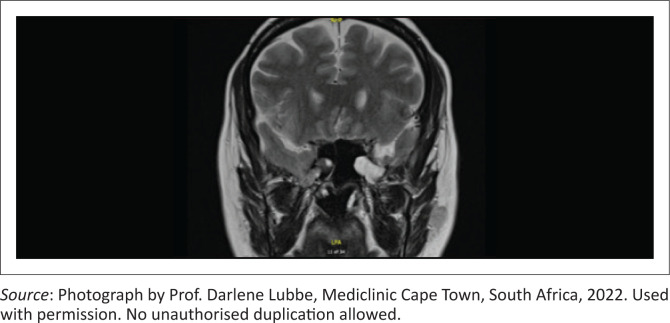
Magnetic resonance imaging scan of paranasal sinus showing isointense encephaloceles in bilateral sphenoid sinuses.
